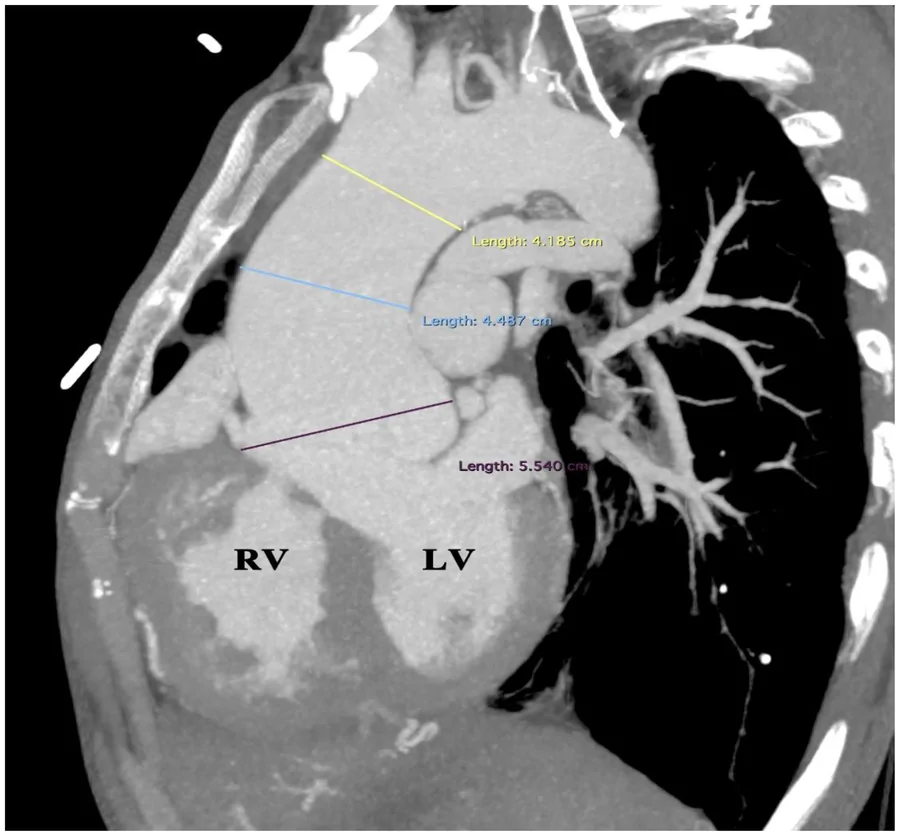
Preoperative computed tomographic angiography demonstrating aortic root dilation (55.4 mm) in the setting of unrepaired tetralogy of Fallot.
